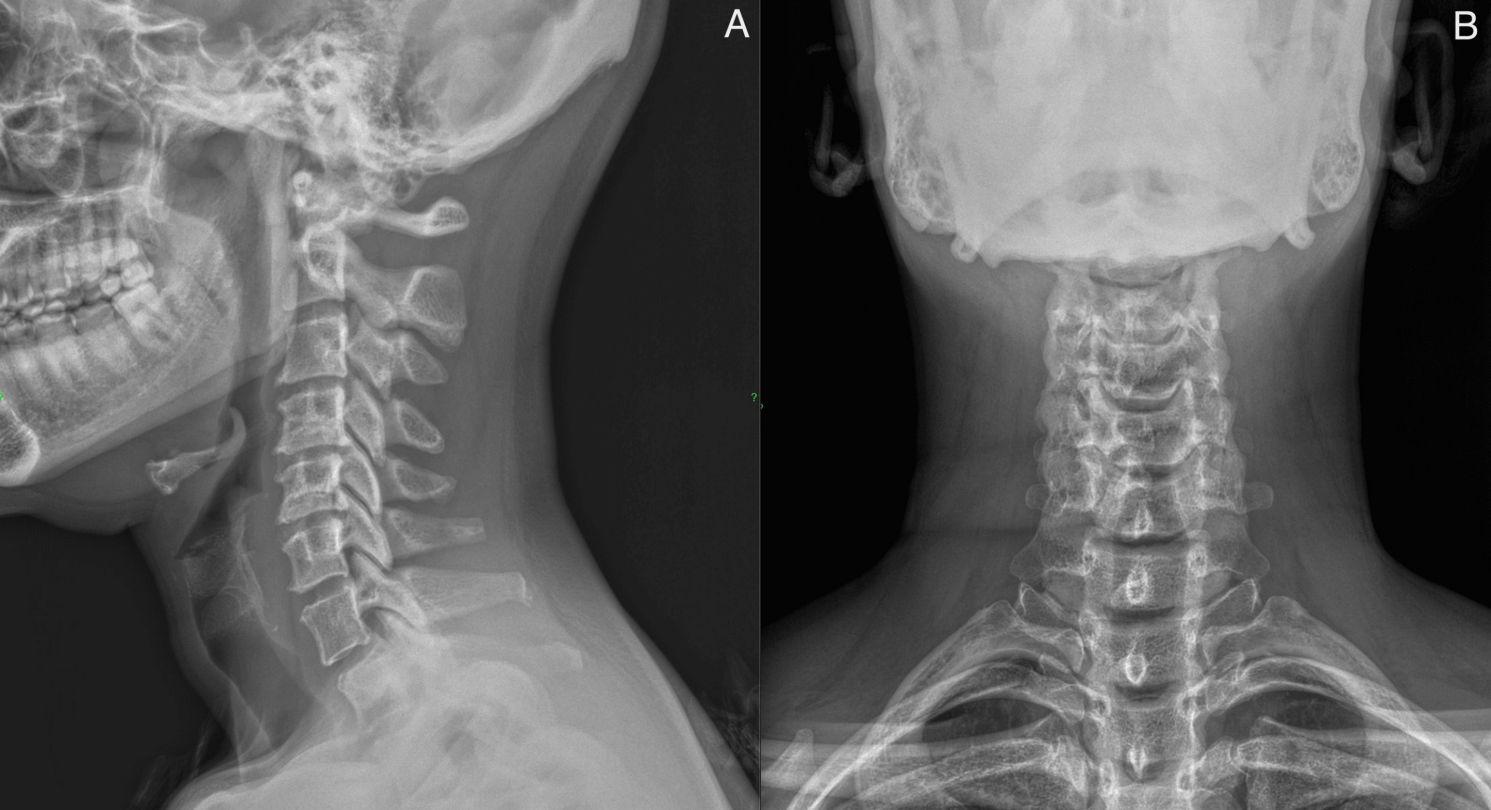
Case C: X-Ray cervical spine, lateral (A) and AP (B) views. There is mild reduction in disc space height at C4-5 and C5-6. Straightening of the cervical lordosis indicative of muscle spasm.
